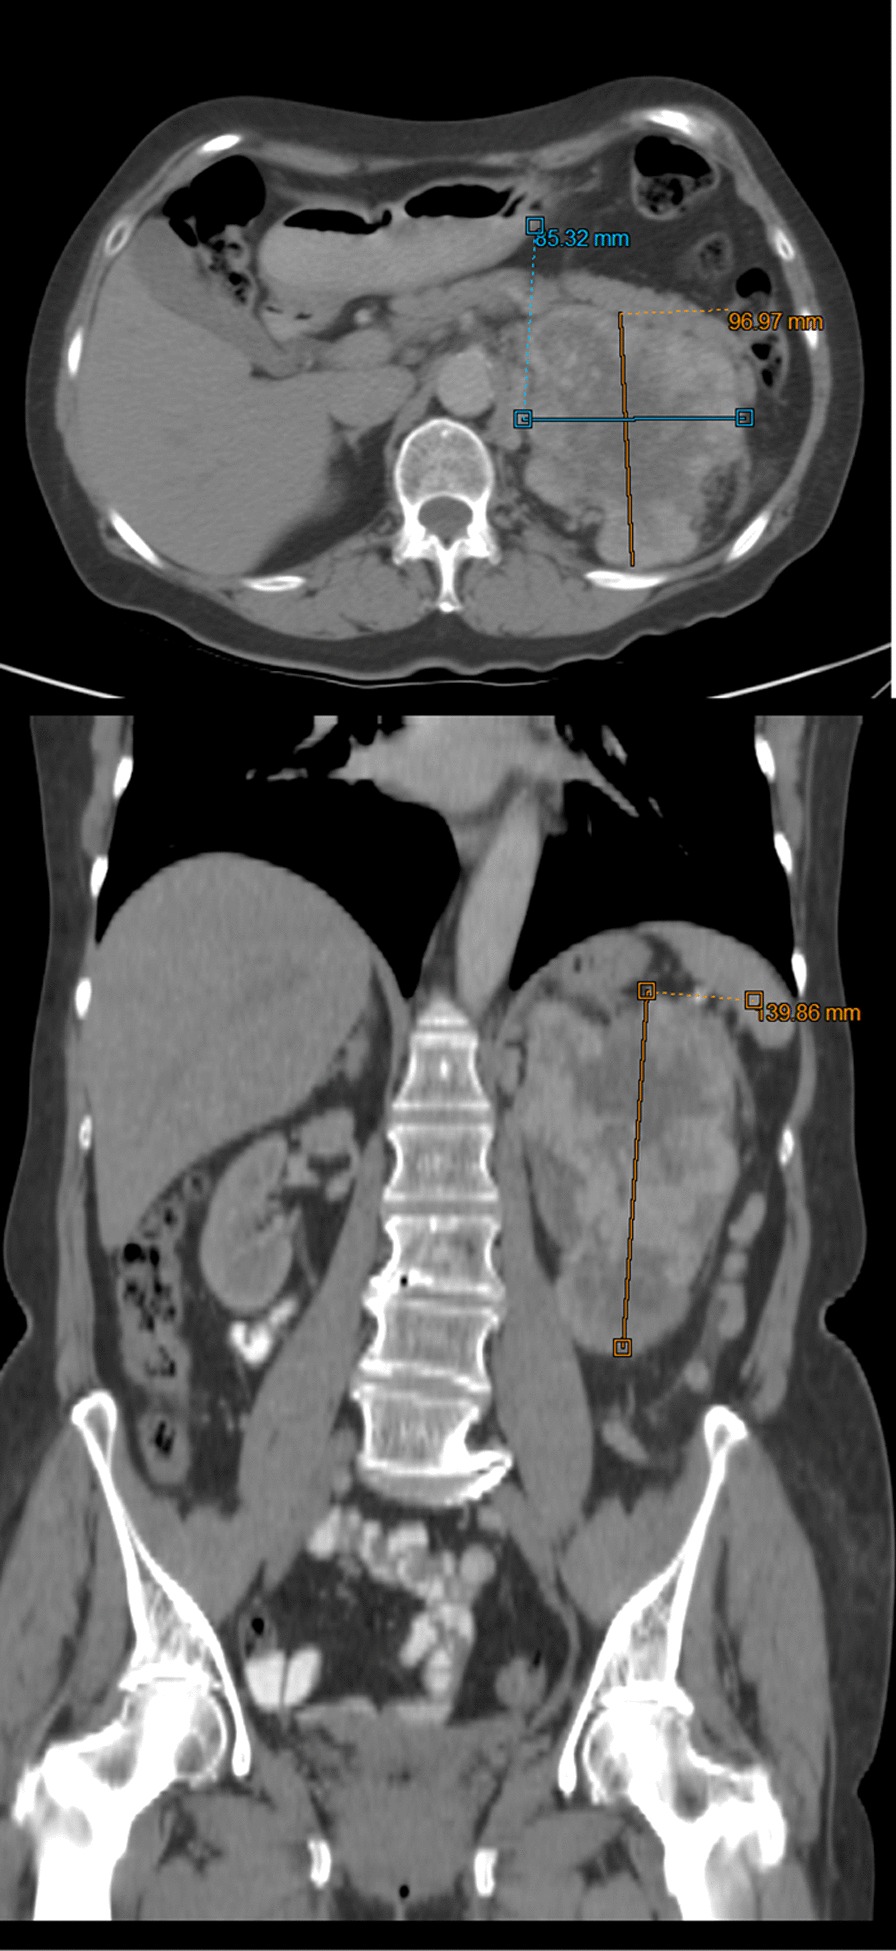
CT scan in which the left kidney cancer is observed with dimensions of 140 × 97 × 85 mm
